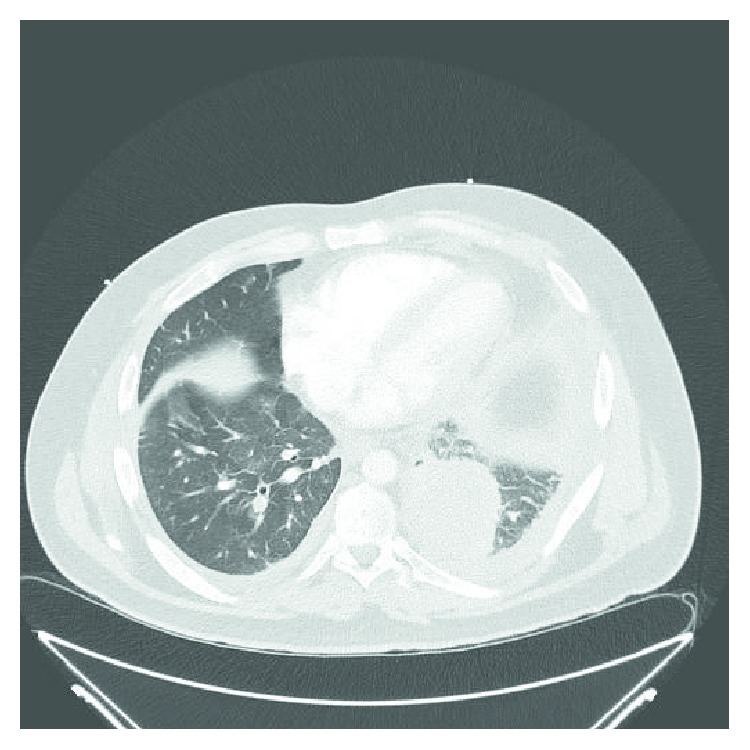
Computed tomography of the chest from June 2017 showing a left lower lobe opacity with preseptal thickening and a small pleural effusion.
